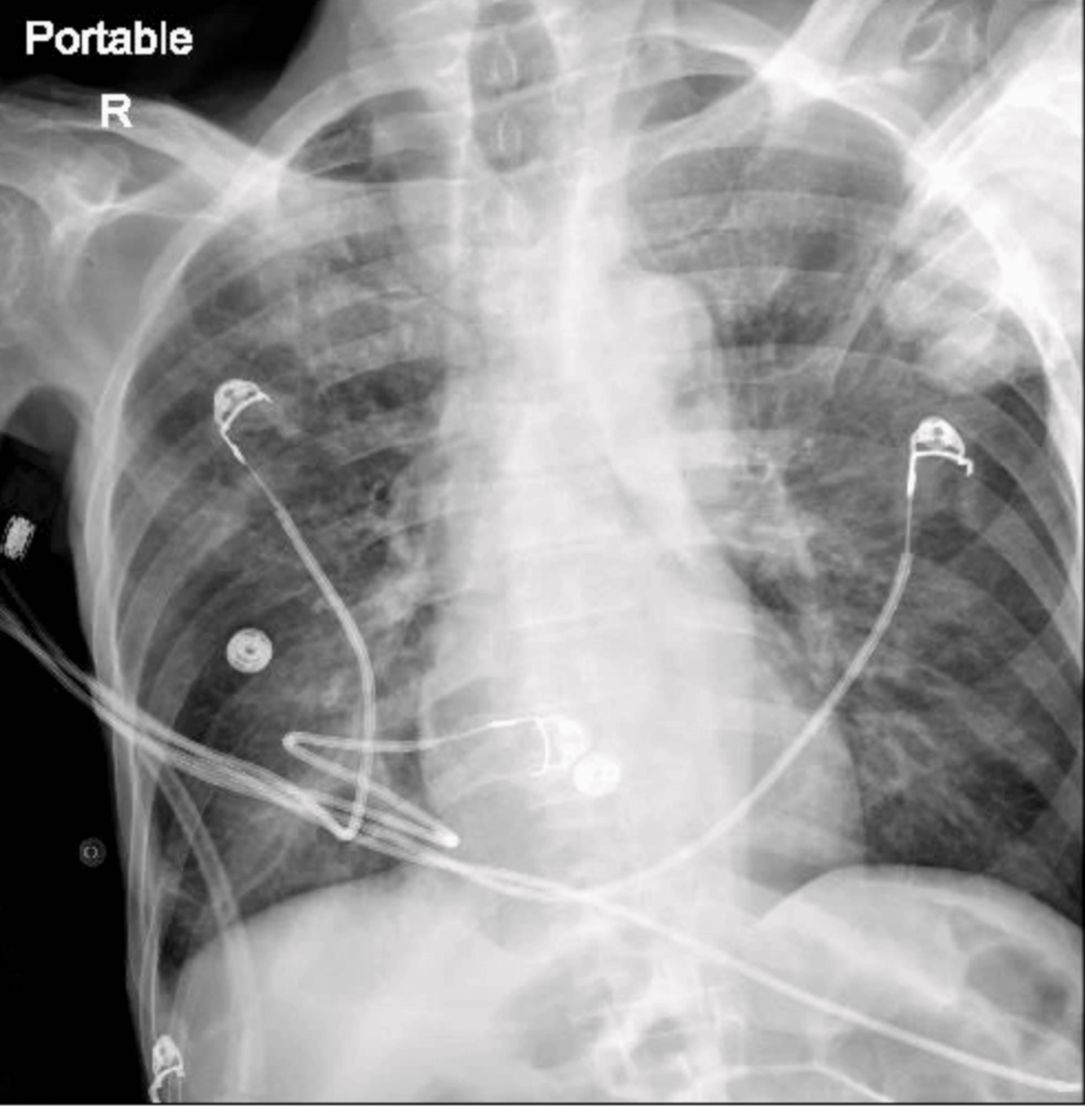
Chest XR on admission showing coarse bronchovascular markings. XR: X-ray
